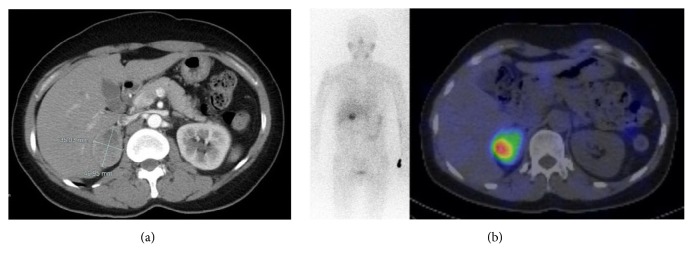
(a) Abdominal computed tomography showing a heterogeneous enhanced mass in the right adrenal gland. (b) Image generated by scintigraphy and single-photon emission computed tomography showing the right adrenal mass and demonstrating increased uptake of metaiodobenzylguanidine.
